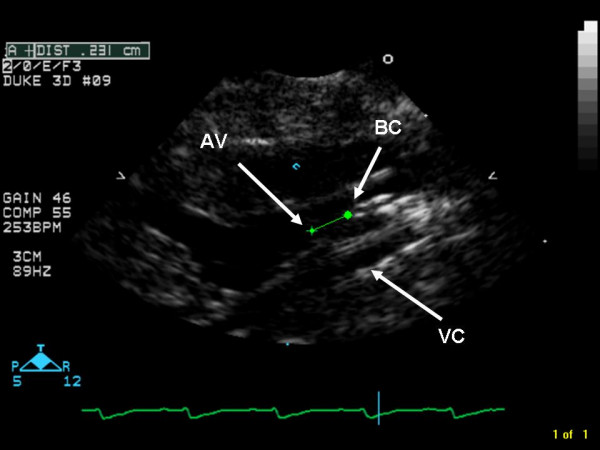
Echocardiographic image of angioplasty balloon catheter positioned near aortic valve. Green distance measure indicating a distance of 0.23 cm from tip of balloon catheter to aortic valve. AV = aortic valve, BC = tip of balloon catheter, VC = multi-orificed venous cannula advanced into right atrium.
